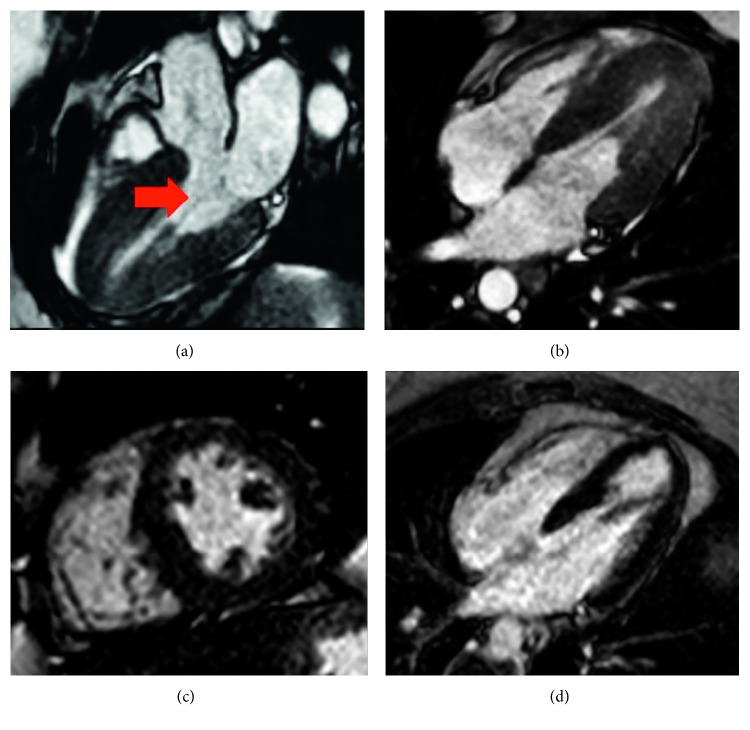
Cardiac MRI showing multiple heads of the posteromedial papillary muscle in the three chamber view (a), four chamber view (b), and short axis view (c), with a suggestion of an abnormal chordal attachment to the anterior mitral valve leaflet (red arrow). Delayed gadolinium imaging (d) showing no increased signal.
